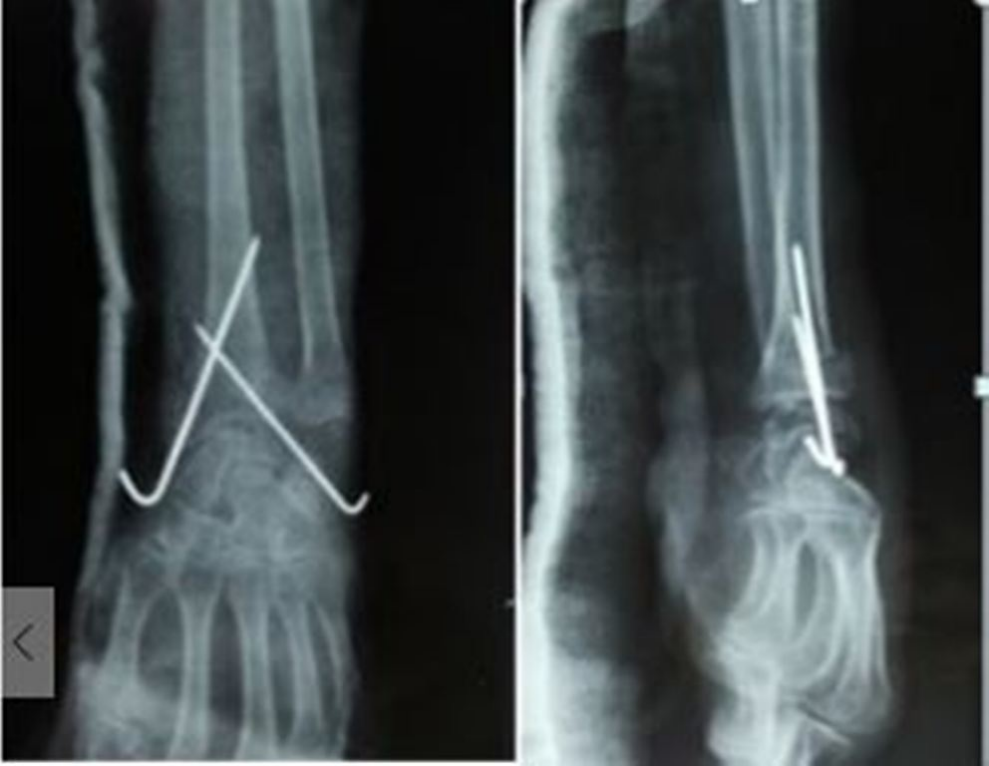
Post-reduction anteroposterior and lateral radiographs showing good reduction
